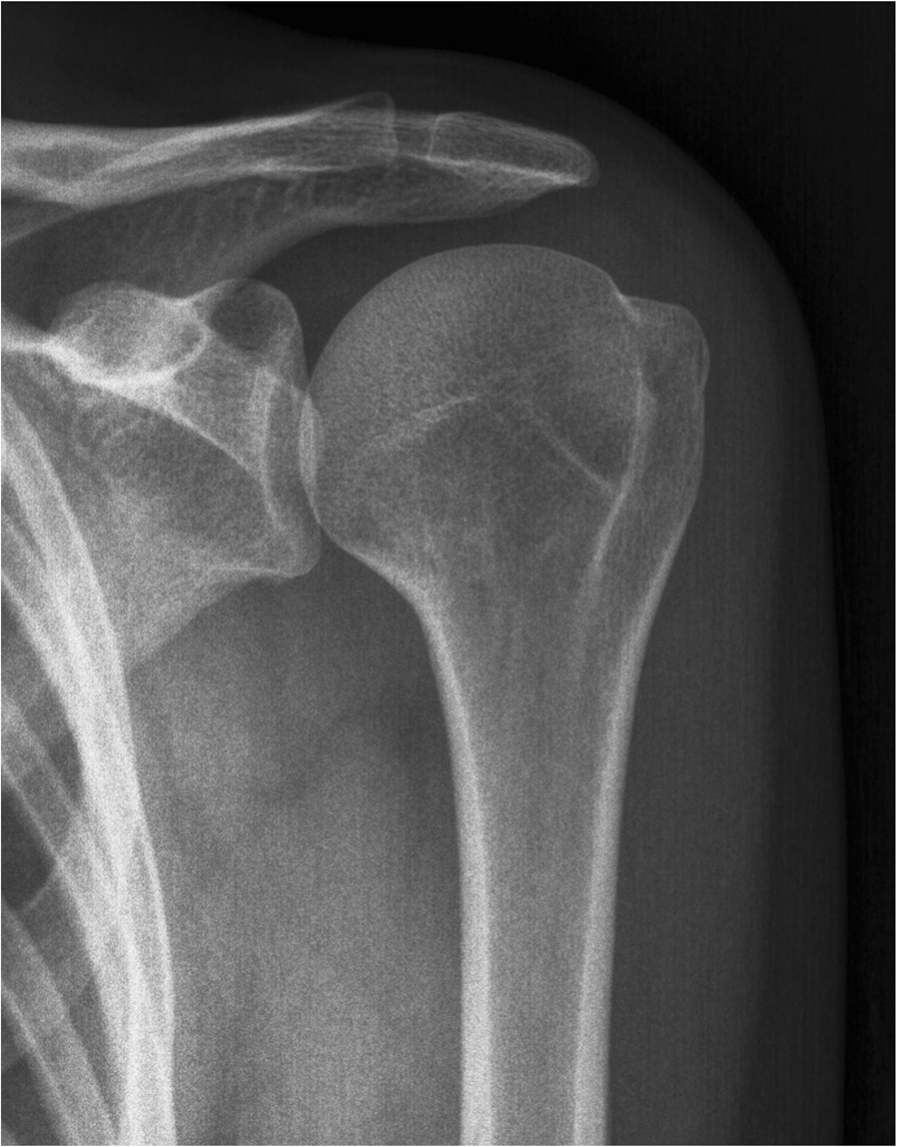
Shoulder radiographs of a patient with undisplaced greater tuberosity fracture
